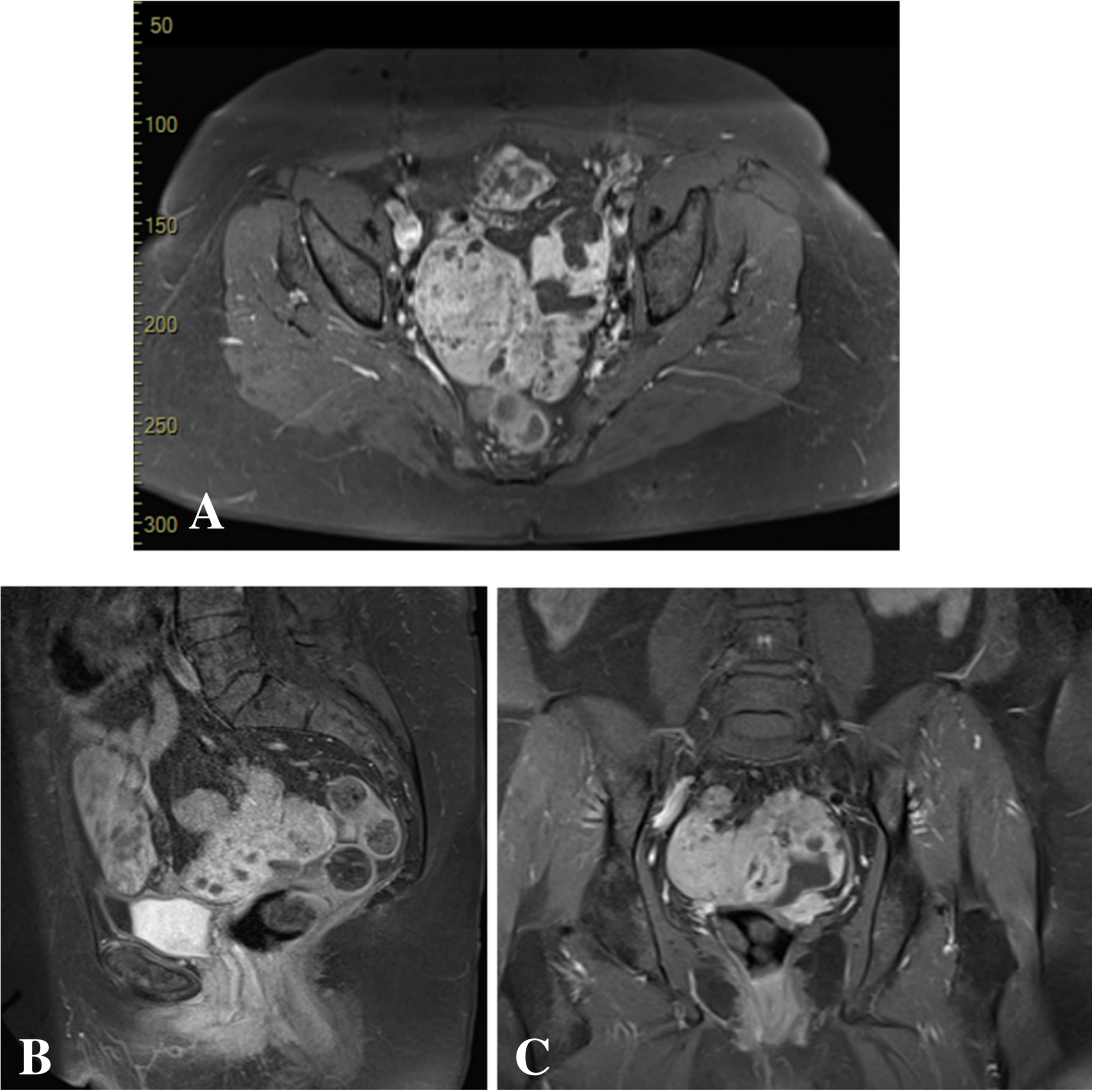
Patient 1 – magnetic resonance images of the recurrence of ovarian granulosa cell tumor. a Axial, b sagittal, and c coronal T2-weighted images showing a local tumor recurrence (87×108×70 mm), diffuse peritoneal metastasis (68×35 mm) with ascites from the pelvis, a parailiacal pathological lymph node (15×10 mm), and an inguinal pathological lymph node (62×32 mm) from the left side of the pelvis
